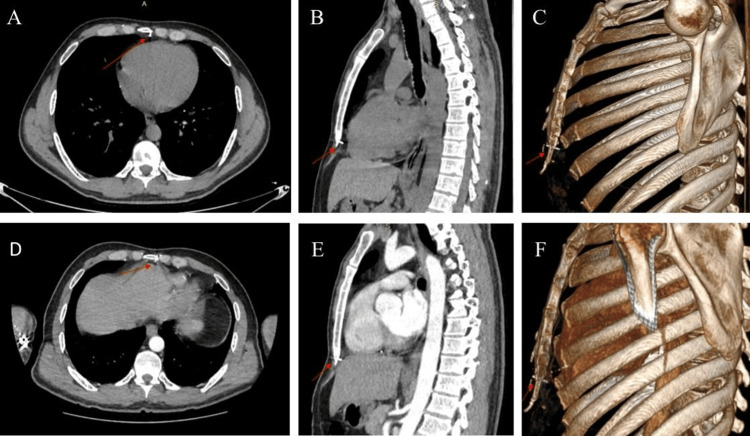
Preoperative thoracic computed tomography evaluation. (A-C) Non-contrast axial, sagittal, and three-dimensional reconstructed computed tomography images demonstrating the metallic nail (red arrows) penetrating the inferior sternum toward the pericardium. (D-F) Contrast-enhanced axial, sagittal, and three-dimensional reconstructed images showing the relationship of the foreign body (red arrows) with cardiac structures. A subtle displacement of the nail is observed between sequences, secondary to respiratory motion.
